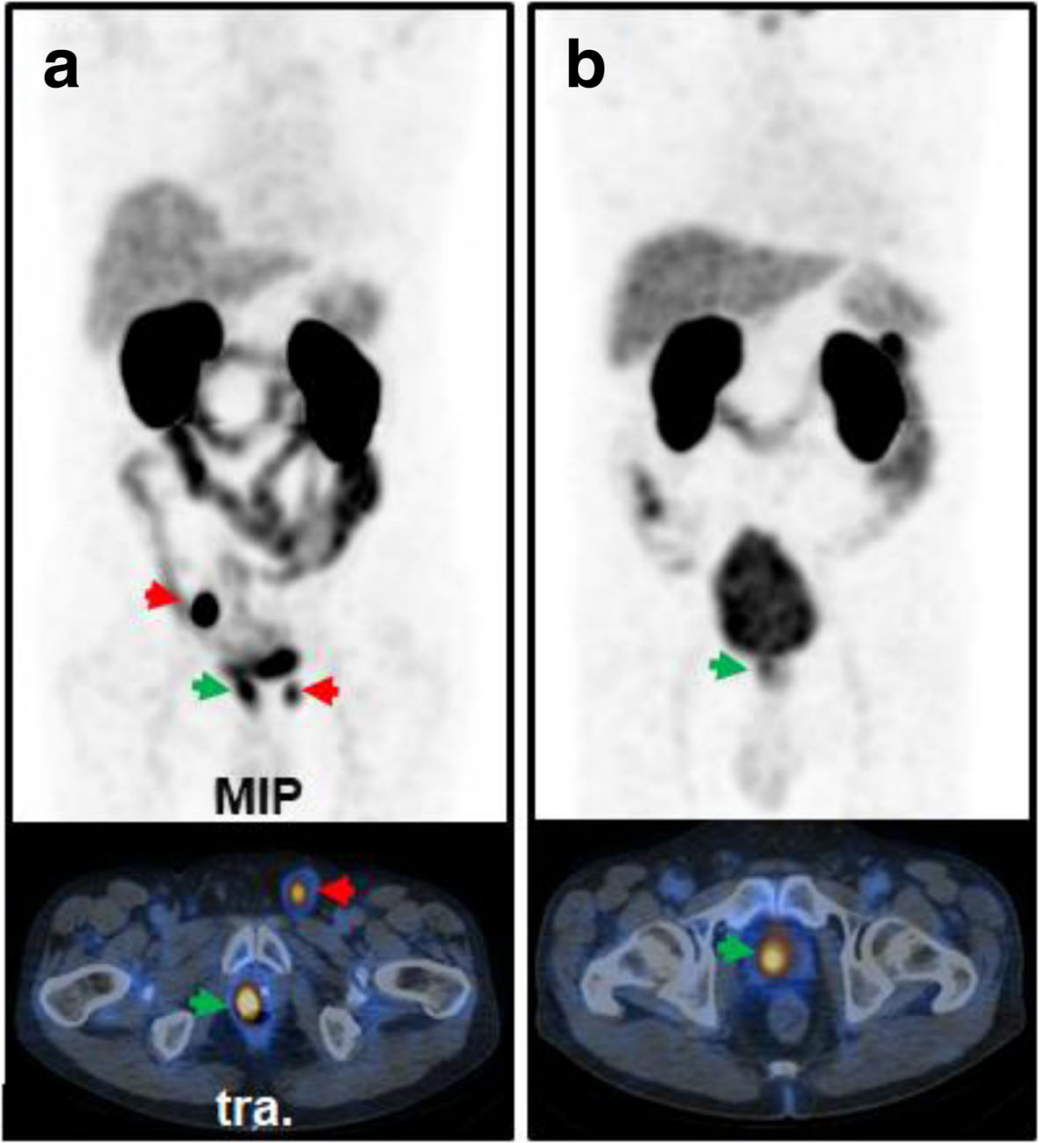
[^99m^Tc]Tc-PSMA-I&S-SPECT/CT in primary high risk PCa. a 71-year-old man with Gleason 9 PCa (PSA 46 ng/ml). Apart from the primary tumor (green arrow), increased PSMA expression was detected in one lymph node in the right iliac region and in the left inguinal region (red arrows)(TxN1M0). b 77-year-old man with Gleason 9 PCa (PSA 80 ng/ml). PSMA expression was increased in the primary tumor only (green arrow), no metastases were detected (TxN0M0)
